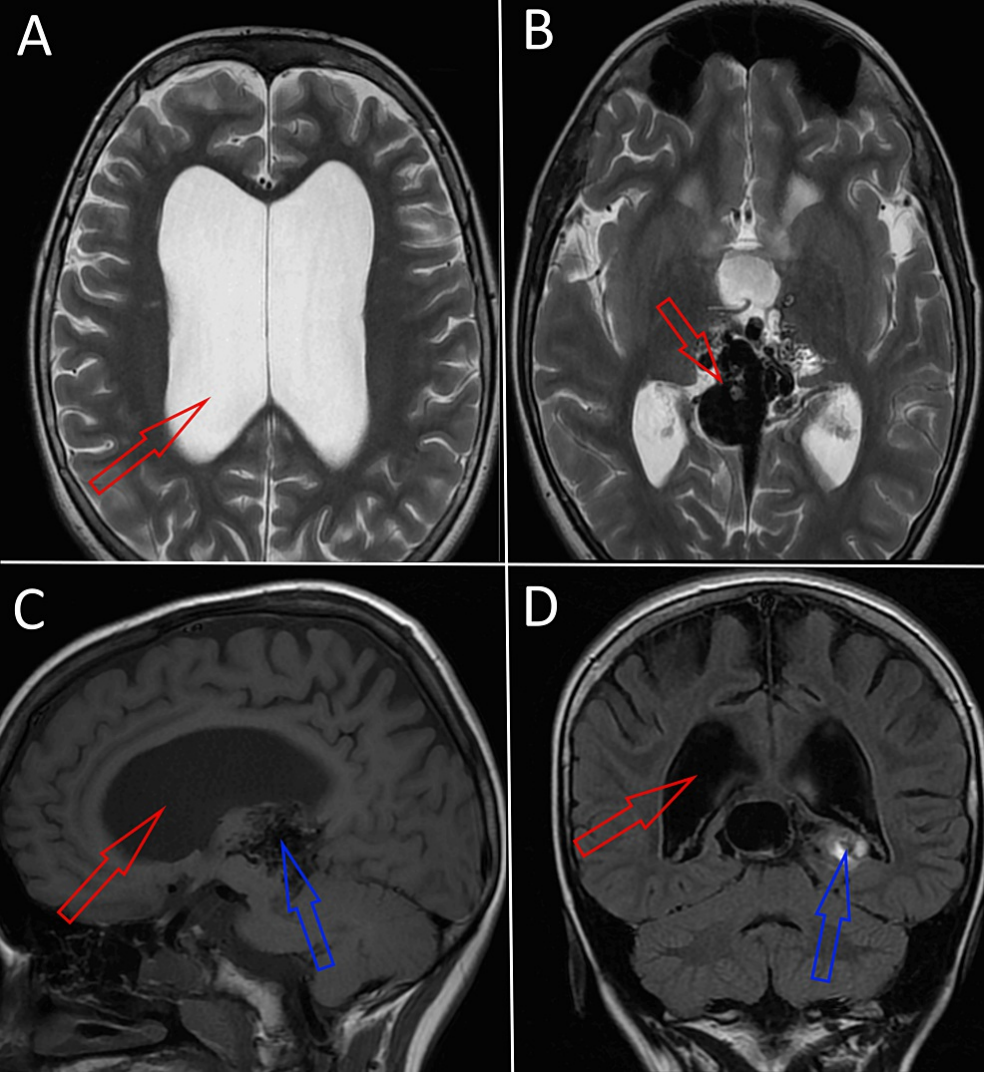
MRI taken two days after birth: (A) T2-weighted image from the axial plane showing abnormally enlarged lateral ventricles due to obstructive hydrocephalus; (B) T2-weighted image from the axial plane at the level of the prosencephalon, revealing the presence of a VGAM; (C) T1-weighted image from the sagittal plane revealing enlarged ventricles (red) and the presence of a VGAM (blue); (D) T1-weighted image from the coronal plane revealing enlarged ventricles (red) and increased blood flow into the VGAM (blue). VGAM: vein of Galen aneurysmal malformation.
